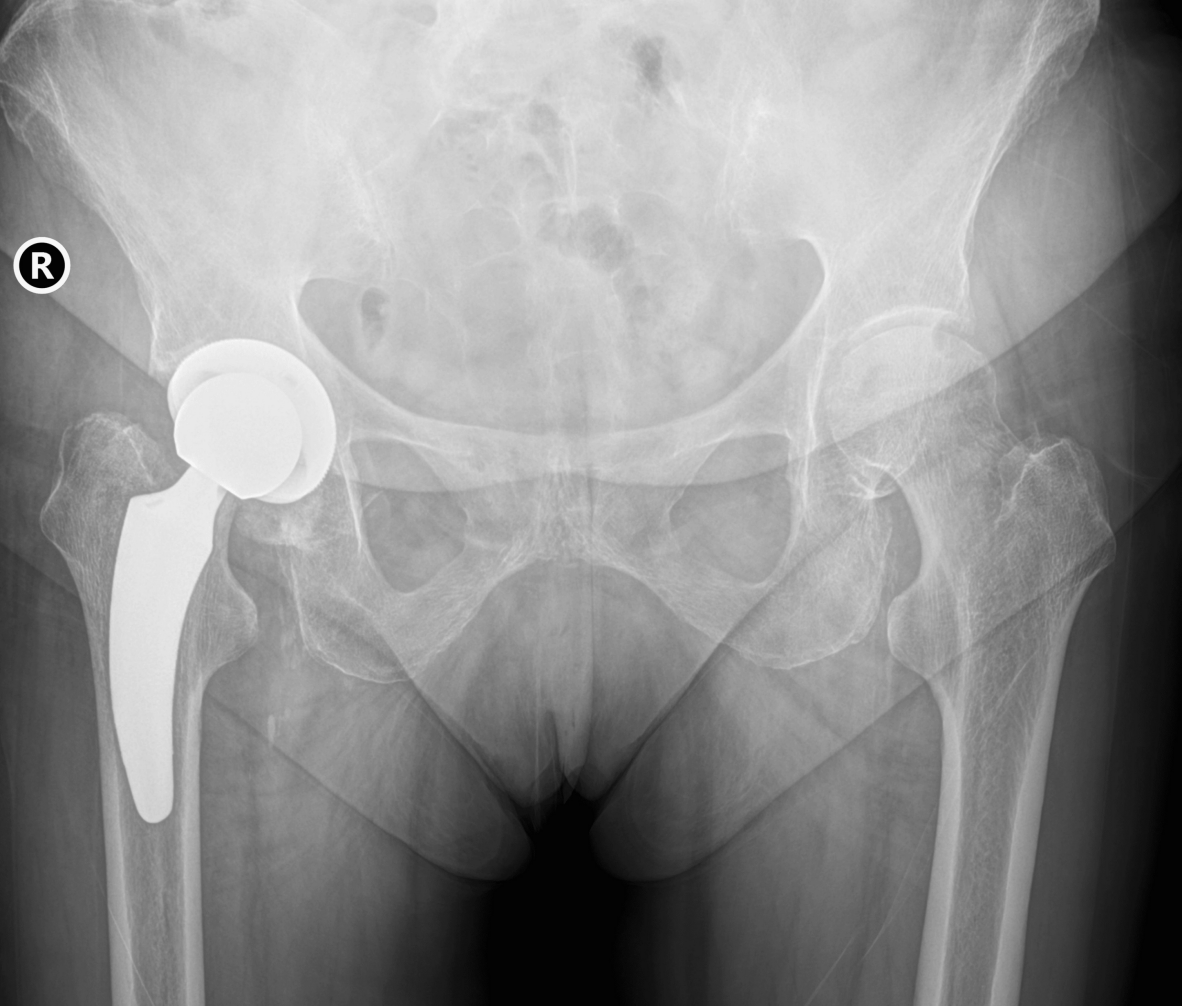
Postoperative radiograph with subsequent subsidence
